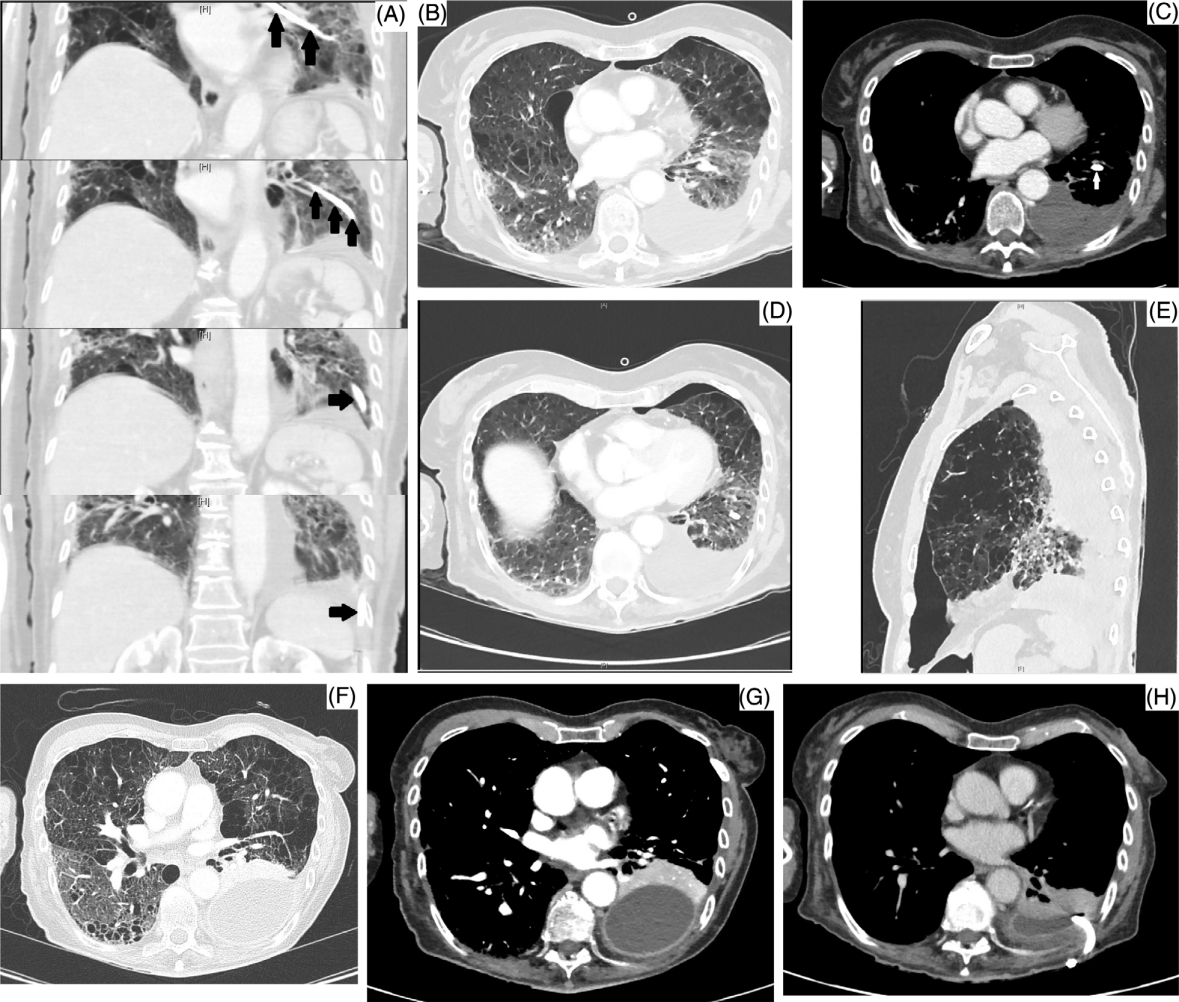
(A‐E) Initial CT Abdomen and subsequent CT Chest on Day 1 of initial empyema. (A) CT Abdomen series showing NGT inserted through left main bronchus tracking down to the base of the lung. (B, C) CT Abdomen showing significant amount of NG feeds in the pleural space at the left lung base. White arrow showing the NGT within the left lower lobe bronchus (Lung view and mediastinal view, respectively). (D, E) Subsequent CT Chest done 3 h later showing progression of pleural effusion and hydropneumothorax following NGT removal. Also noted is the significant amount of emphysema throughout both lung fields. (F, G) CT Chest showing 2nd recurrence of empyema (Lung view and mediastinal view, respectively). (H) CT Chest showing post drainage and intrapleural fibrinolysis improvement of 2nd empyema
